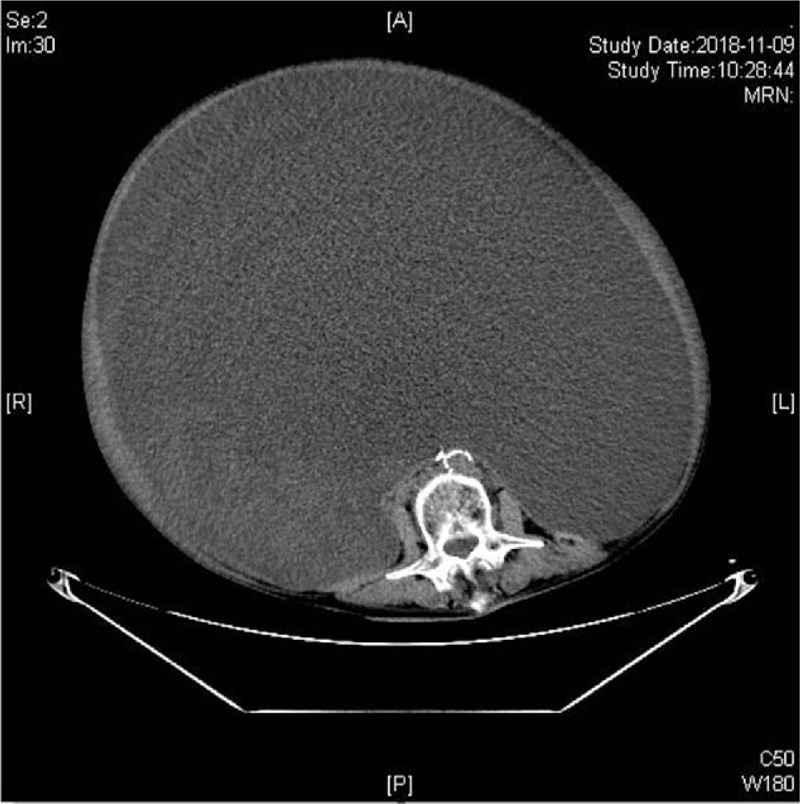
Preoperative abdominal CT shows a giant cystic tumor.
